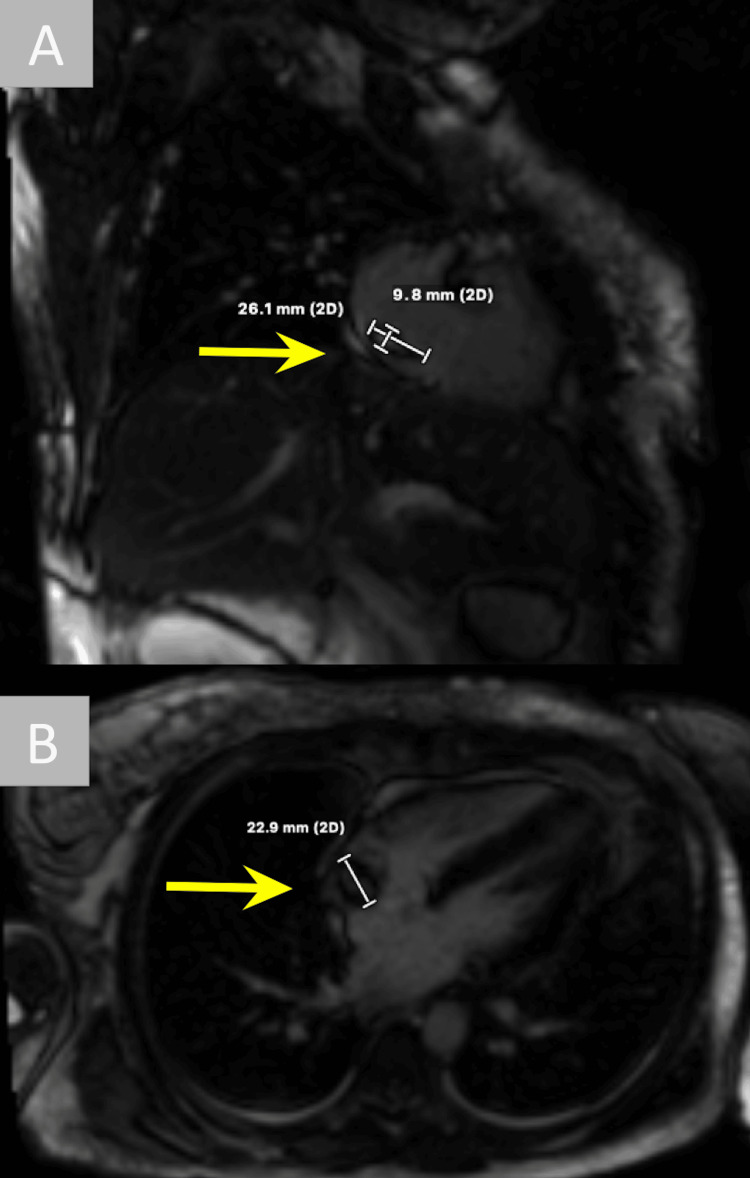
Cardiac magnetic resonance imaging (cMRI) images Cardiac magnetic resonance imaging (cMRI) revealed a right atrial peripherally hypointense lesion measuring 2.6 cm x 2.3 cm x 0.9 cm, with a gray etched core close to the crista terminalis and homogeneous low signal.
